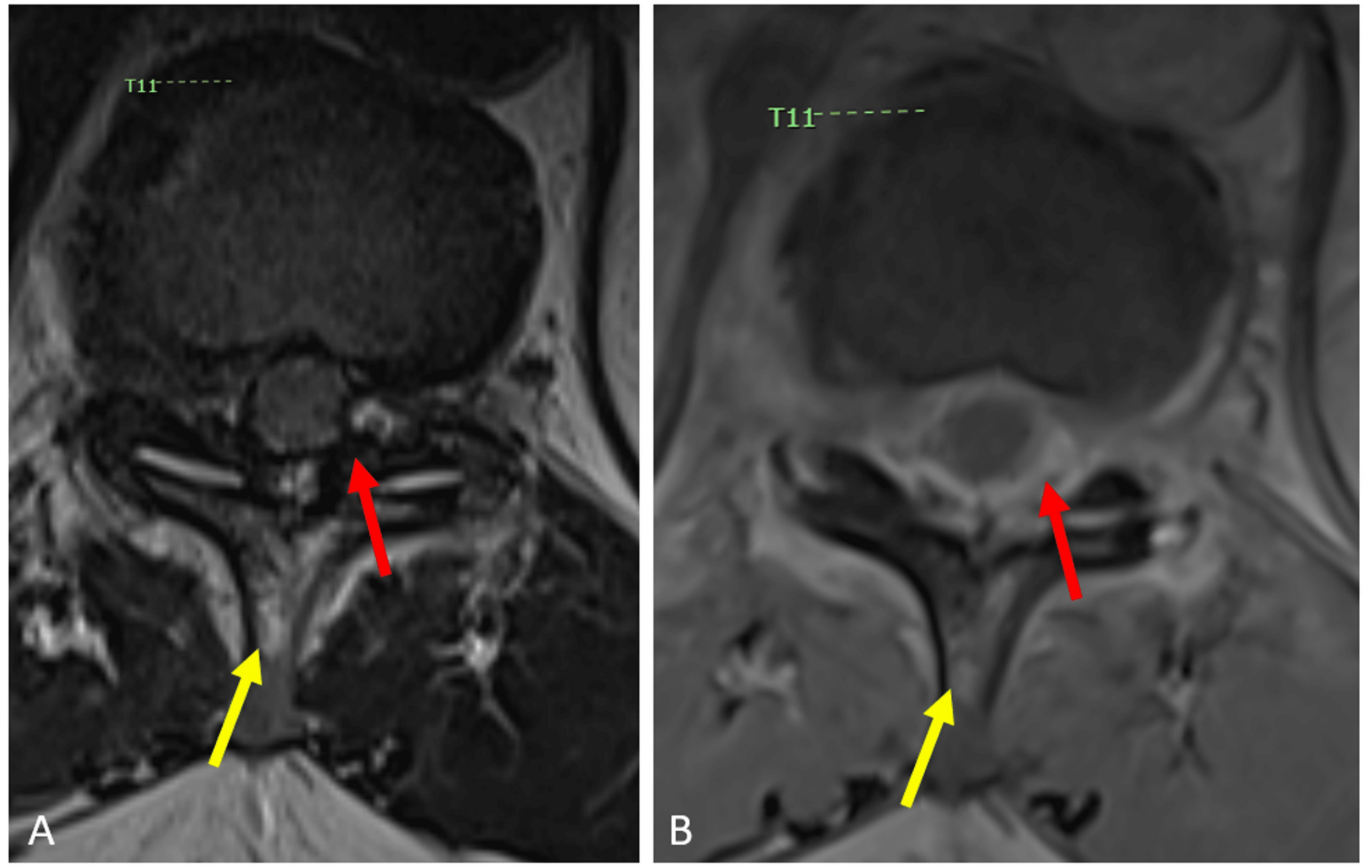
Axial MRI of the Thoracic Spine With and Without Contrast Axial MRI images with T2 (A) and T1 post-contrast fat saturation (B) of the thoracic spine at the level of T11. There is a heterogenous collection that demonstrates avid enhancement in the posterior epidural space causing severe spinal canal stenosis with effacement of the thecal sac (red arrow). There is a T2 hyperintense signal with corresponding contrast enhancement within the T11 spinous process (yellow arrow).
